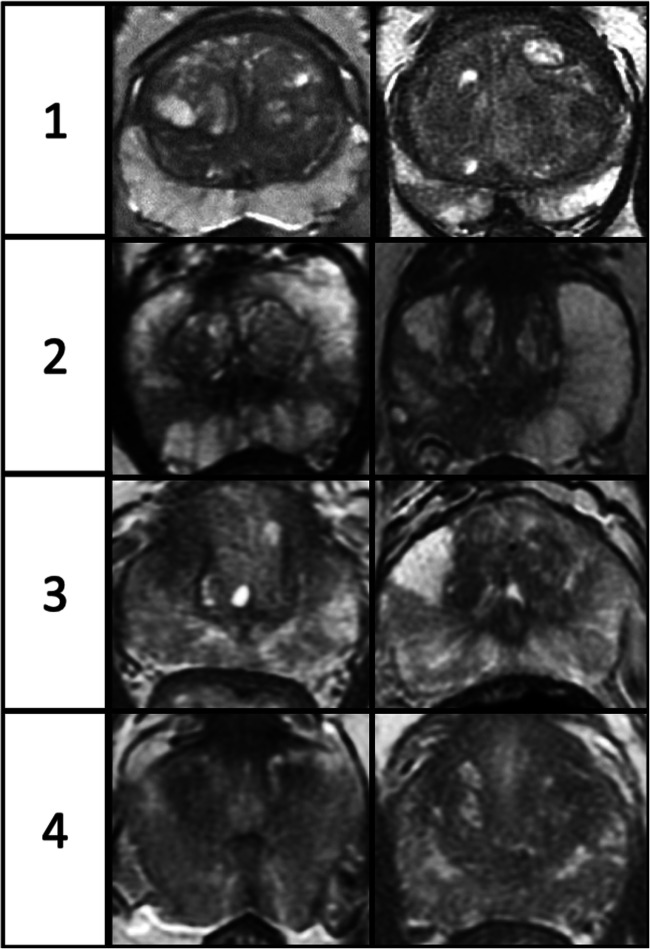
Image examples of T2-weighted imaging scores 1 to 4. Detailed descriptors can be found in Table 1
